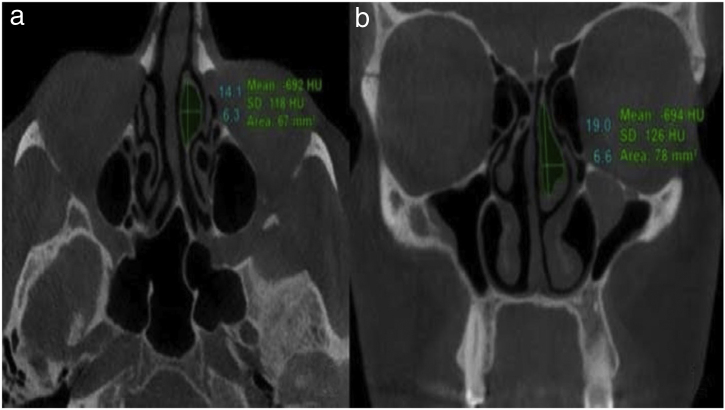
Cone-beam computed tomography images demonstrating (a) anterior-posterior and medio-lateral dimensions as well as overall area in axial plane and (b) height and medio-lateral dimensions in coronal plane.
